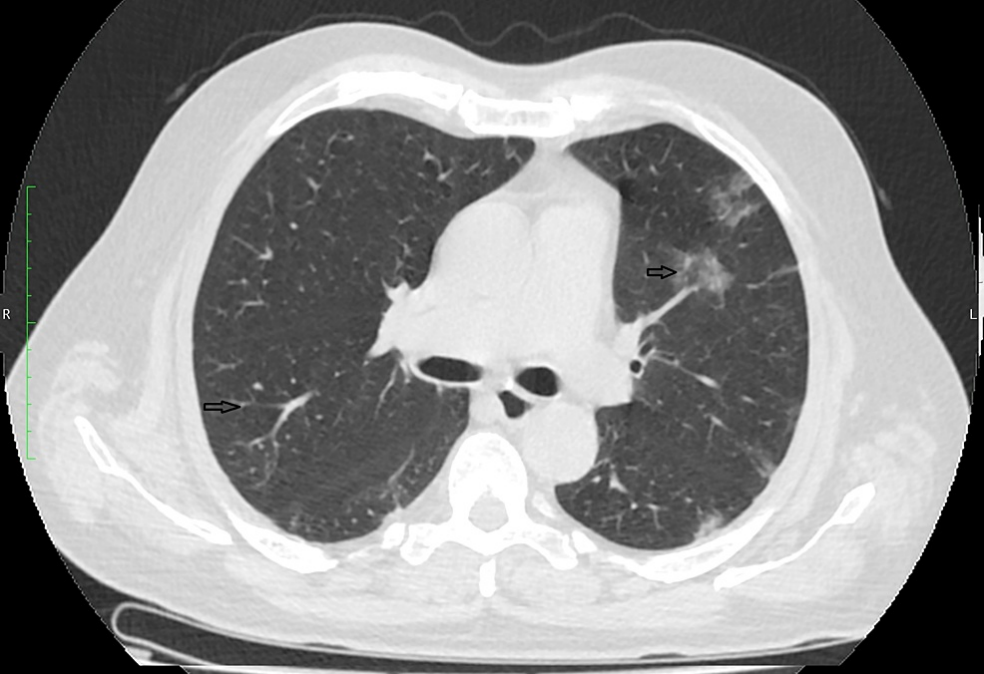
CT Chest Showing Small, Localized Ground-Glass Opacities in the Lung Peripheries
